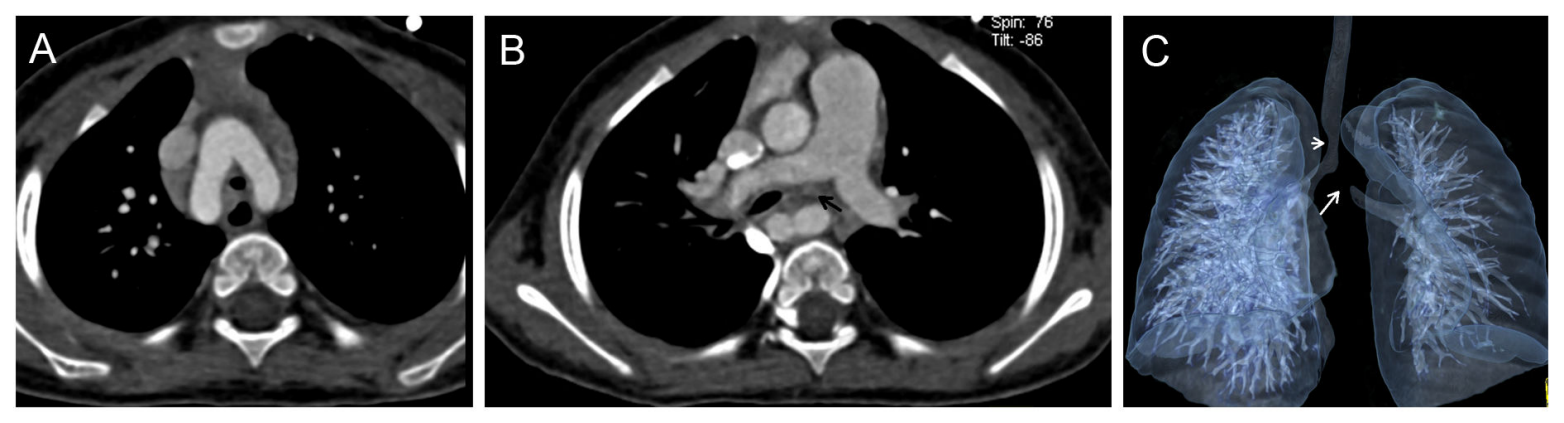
A 21-month boy with double aortic arch and subsequent tracheobronchial narrowing. In addition, a nonvascular left main bronchus stenosis was detected. A. CT axial image demonstrated double aortic arch which caused encirclement of the trachea. B. CT axial image showed the soft issue lesion compressed the left main bronchus, and local lumen was narrow (black arrow). C. Volume Rendering (VR) show the one tracheal stenosisi (short white arrow) caused by double aortic arch and the other severe bronchial narrowing (long white arrow) due to a nonvascular compression.
